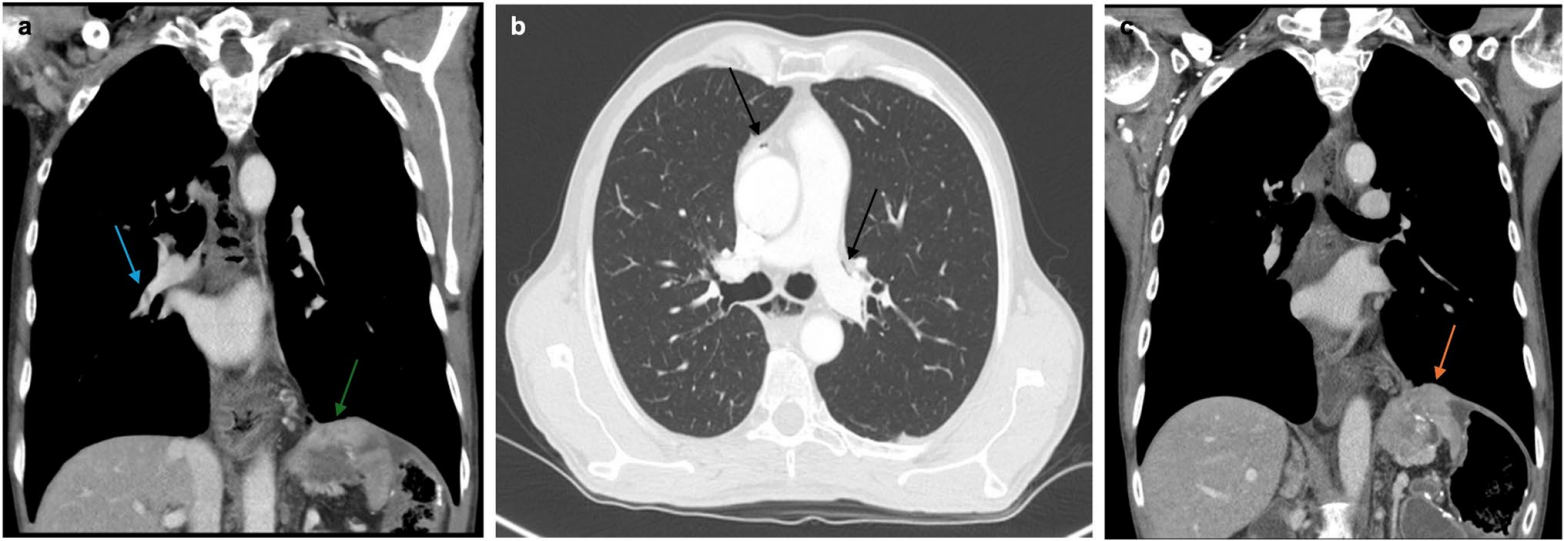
Contrast-enhanced CT scans of the chest after initiation of an anti-angiogenic agent (a) demonstrates a right lower lobe pulmonary embolus (blue arrow) with a 32 mm enhancing lesion in the GE junction (green arrow). Around the same time, another CT (b) showed pneumomediastinum (black arrows). One month after stopping the anti-angiogenic agent, repeat CT (c) showed resolution of the right lower lobe pulmonary embolus and pneumomediastinum with rapid interval growth in the size of the lesion (orange arrow) to 48 mm. Timeline demonstrates the balance between treatment related adverse side effects and treatment of the primary malignancy
